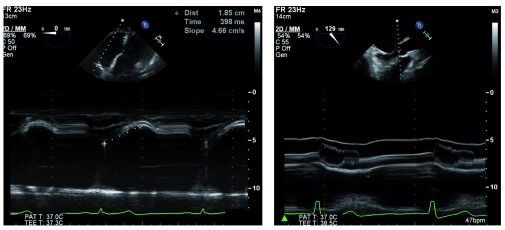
M-mode echocardiography is available on most modern transesophageal echocardiography probes. It is clinically useful for diagnosing and characterizing many clinical conditions, such as right ventricular function, by measuring tricuspid annular plane systolic excursion (left) or assessing dynamic left ventricular outflow tract obstruction hallmarked by fluttering and premature closure of the aortic valve leaflets (right). 2D, two-dimensional; bpm, beats per minute; MM, M-mode; PAT T, patient temperature; TEE T, transesophageal echocardiography temperature.
